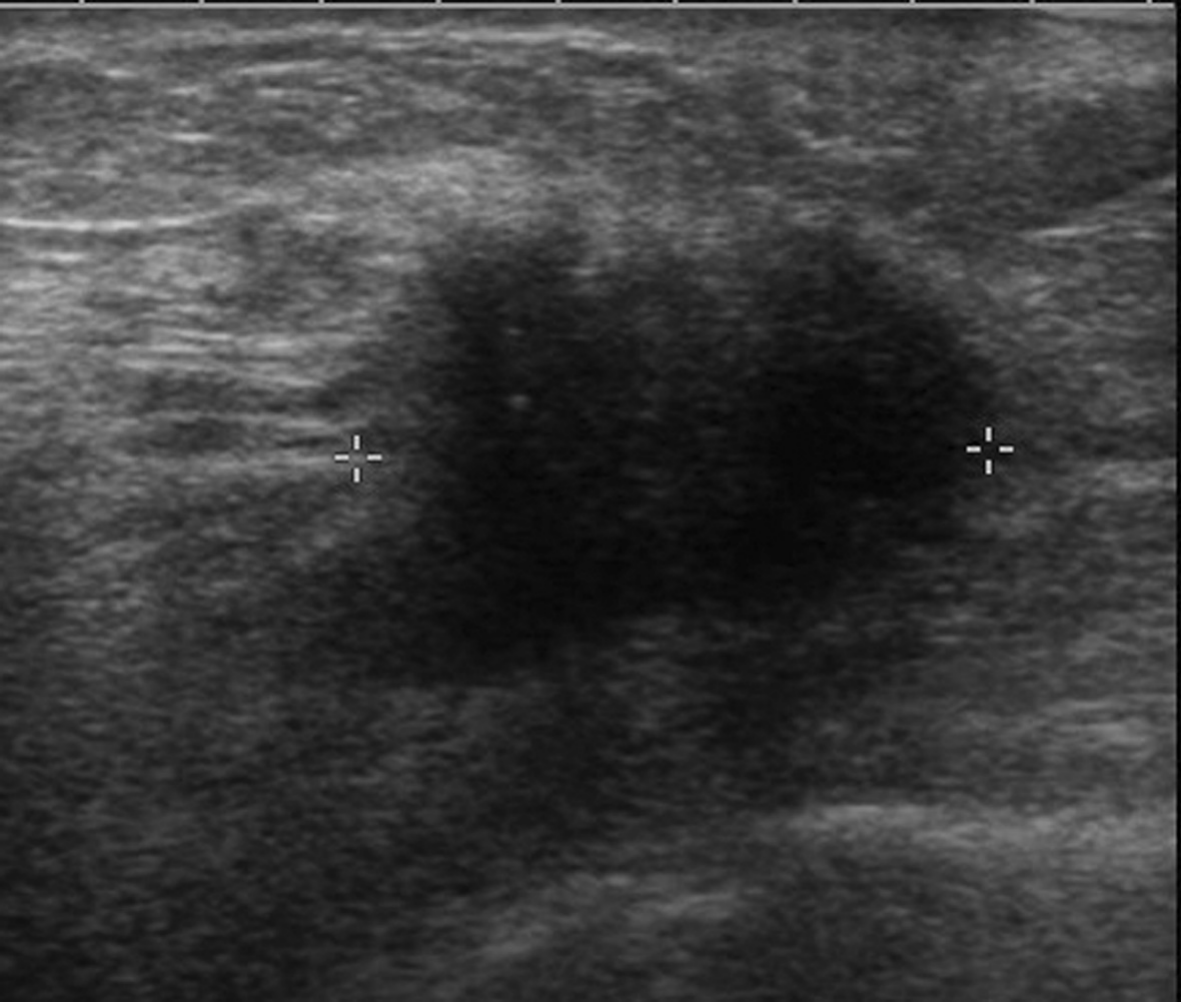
Multifocal invasive ductal carcinoma in a 53-year-old woman with dense breasts. The ultrasound image clearly demonstrates a 26-mm hypoechoic mass with irregular margins highly suggestive of malignancy.
